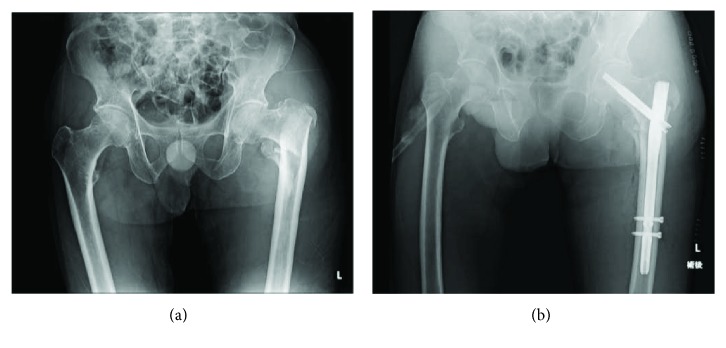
Preoperative (a) and immediate postoperative (b) radiographs show stable intertrochanteric fracture without comminution, acceptable reduction, and good positioning of the nail and screws.
